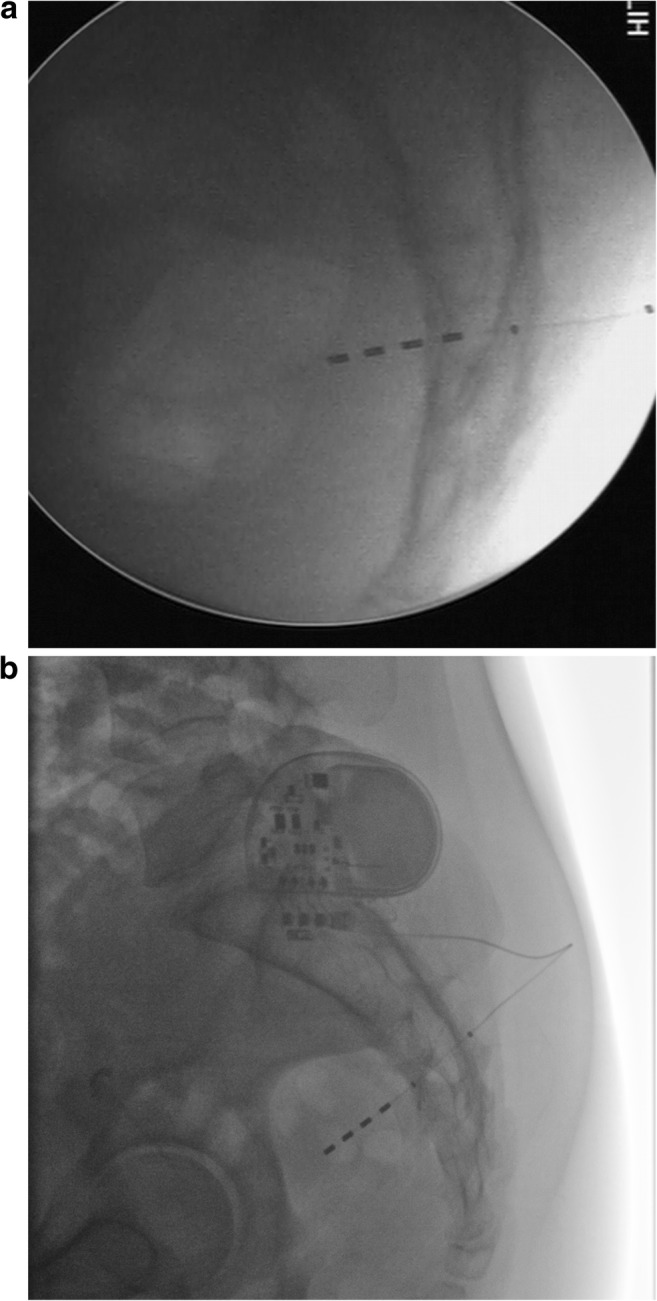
a Lateral pelvic radiography showing electrode position at implantation and b after loss of efficacy
